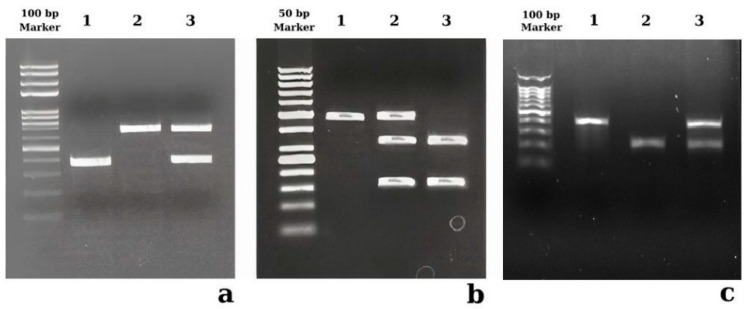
The restriction enzyme patterns of ACE rs4646994 (a), PPRGC1A rs8192678 (b), and ACTN3 rs1815739 (c) polymorphisms. (a) The first lane is a 100 bp size marker, lane 1 is del/del (homozygous), lane 2 is ins/ins (homozygous), and lane 3 is ins/del (heterozygous). (b) The first lane is a 50 bp size marker, lane 1 is TT (homozygous), lane 2 is TC (heterozygous), and lane 3 is CC (homozygous). (c) The first lane is a 100 bp size marker, lane 1 is CC (homozygous), lane 2 is TT (homozygous), and lane 3 is CT (heterozygous).
